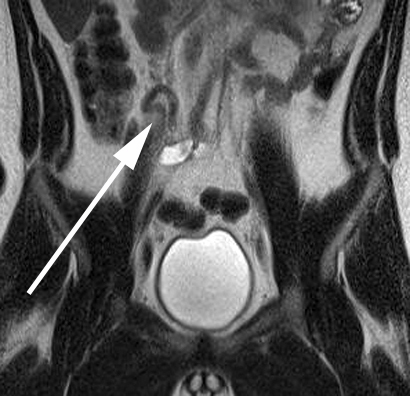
A 17-year-old male with abdominal pain. Coronal *T_2_* weighted MR image without fat saturation demonstrates a normal caliber appendix adjacent to the cecum, draping over the iliac vessels. No fluid or edema is seen surrounding the appendix, and there is no evidence of mural thickening. As on the CT image of the normal appendix, it is coiled upon itself as opposed to the taut morphology seen in an inflamed appendix.
